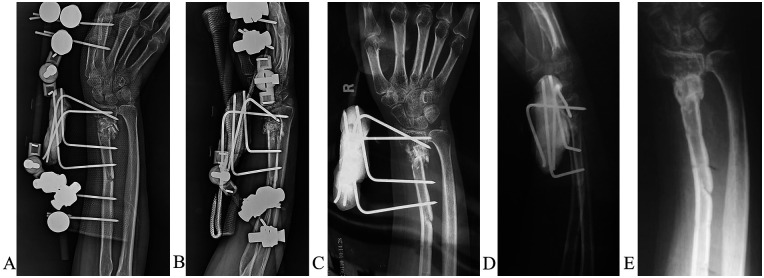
(A) A PA X-ray taken immediately after surgery. (B) A lateral X-ray. (C) Bone healing 1.5 months after surgery on an anteroposterior X-ray. (D) A lateral X-ray. (E) A PA X-ray taken after 2 years.
